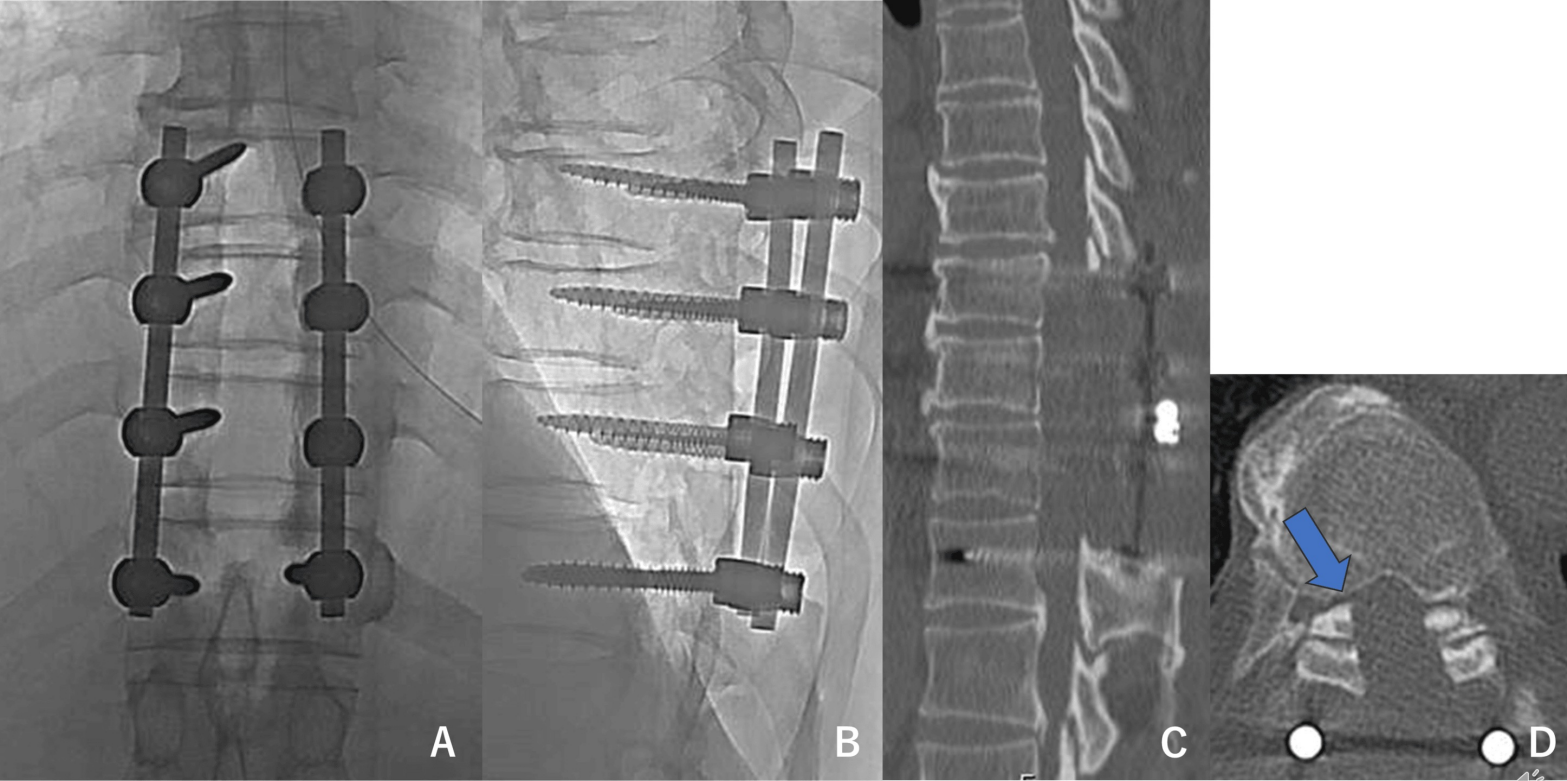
Postoperative X-ray and computed tomography (CT). (A) X-ray: anteroposterior view. (B) X-ray: lateral view. (C) Sagittal CT. (D) Axial CT: all ossifications of the ligamentum flavum were resected (arrow).
